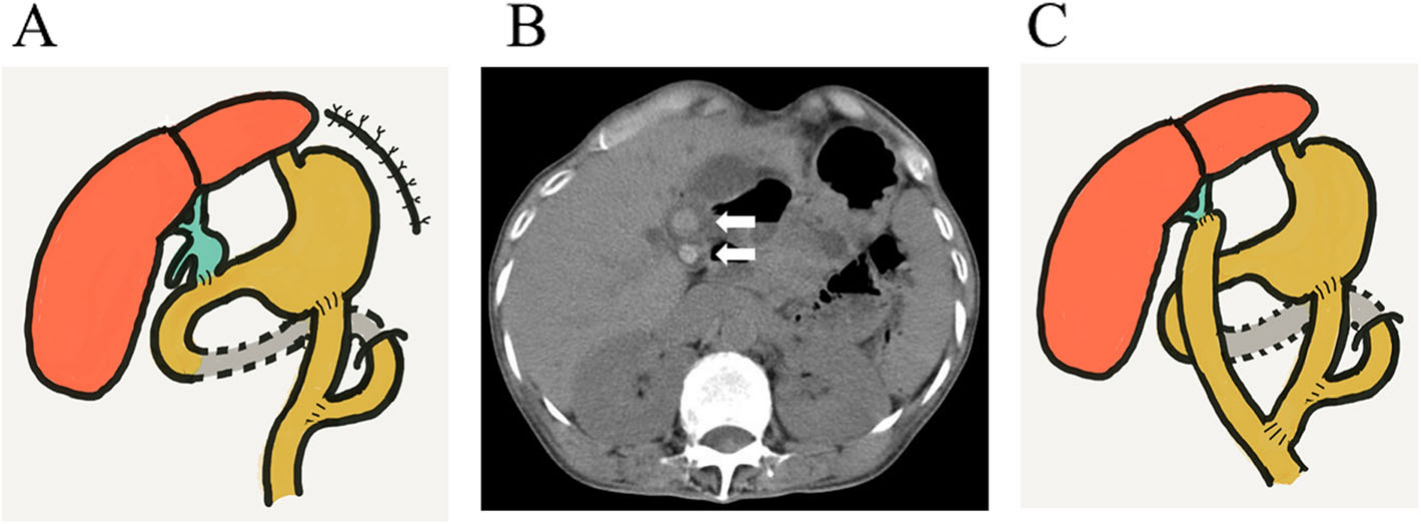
a The gastrojejunostomy was restored in a Roux-en-Y fashion at 31 years of age. Diaphragmatic plication was also performed. b Computed tomography scan shows gallstones at the porta hepatis at 54 years of age. White arrows: gallstones. c The cystic bile duct and gallbladder were resected. Additionally, biliary reconstruction was performed by hepaticojejunostomy in a Roux-en-Y fashion
